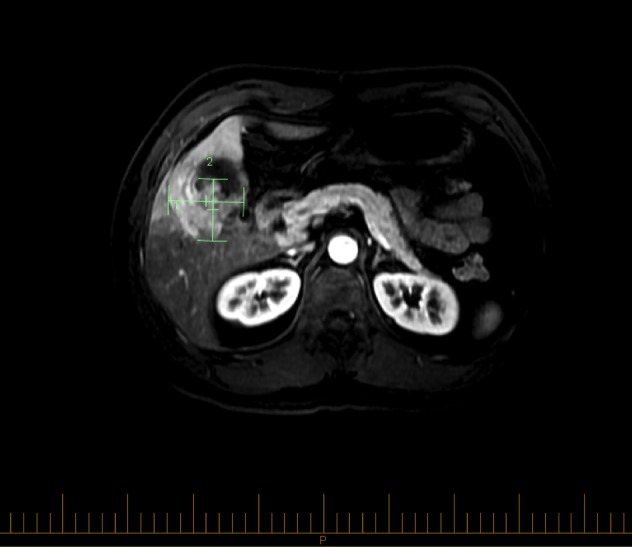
MRI with gadolinium prior to initial surgical exploration A posterolateral gallbladder mass extending to the abdominal wall muscle and liver.
